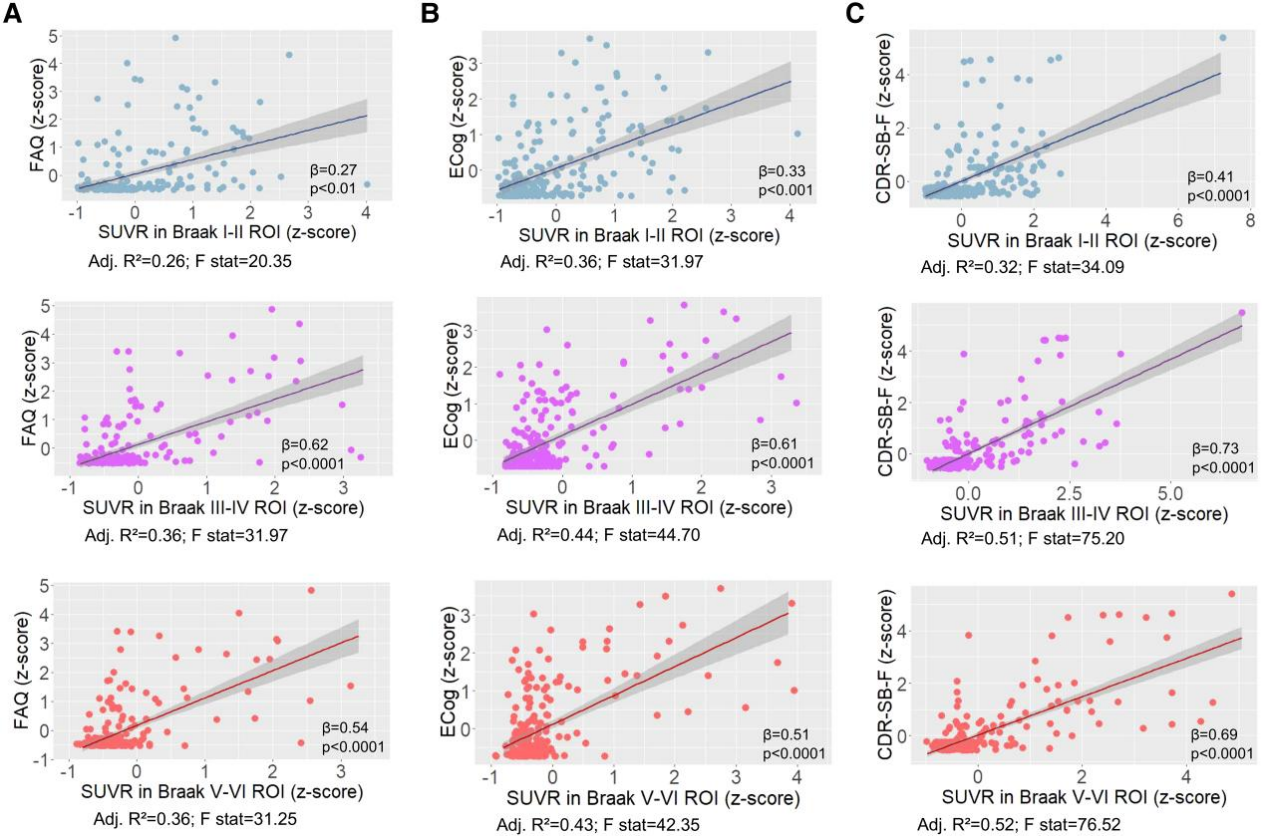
Association between the SUVR in Braak ROIs and functional scores at baseline. The graphs depict the association between tau-PET SUVR in Braak regions and functional performance as assessed by the FAQ (A), the ECog (B), and the CDR-SB-F (C). Depicted *β*-coefficients and *P*-values were obtained in linear regression models adjusted for age, sex and neocortical [^18^F]AZD4694 Aꞵ PET SUVR. CDR-SB-F, functional Clinical Dementia Rating sum of boxes; ECog, Everyday Cognition; FAQ, Functional Activities Questionnaire; ROI, region of interest; SUVR, standardized uptake value ratio.
